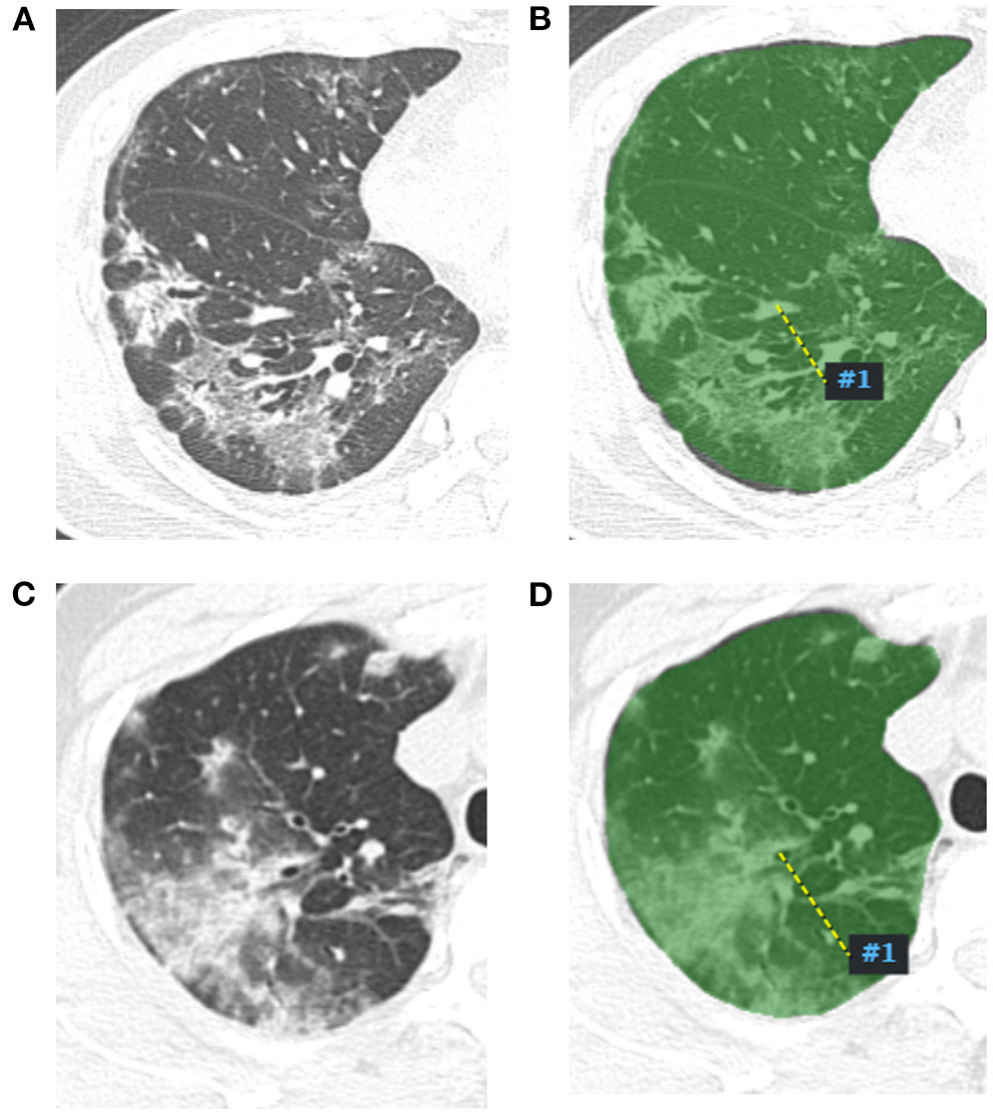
Radiomics features extract map of pneumonia lesion. A two-dimensional image of the largest pneumonia lesion area of a COVID-19 patient in the right lung (A), the lesion presented as blurred patchy ground glass opacity with little consolidation. The region of interest of the largest pneumonia lesion area was manually delineated in the right lung image, which is displayed as the green area (B), and artificial intelligence software automatically extracted the radiomics features and output from a computer. A two-dimensional image of the largest pneumonia lesion area of an influenza viral pneumonia patient in the right lung (C), the lesion presented as ground glass opacity with some consolidation; the CT manifestation was similar with the COVID-19 pneumonia; we used the same method to extract radiomics features from the image (D).
